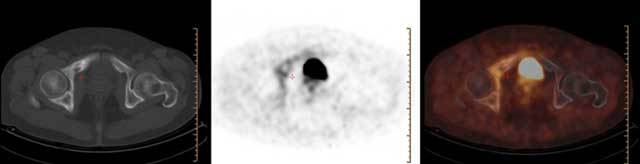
The axial-fused ^68^Ga- DOTANOC PET/CT images show increased uptake in the right pubic bone, superior pubic ramus, and acetabulum.
